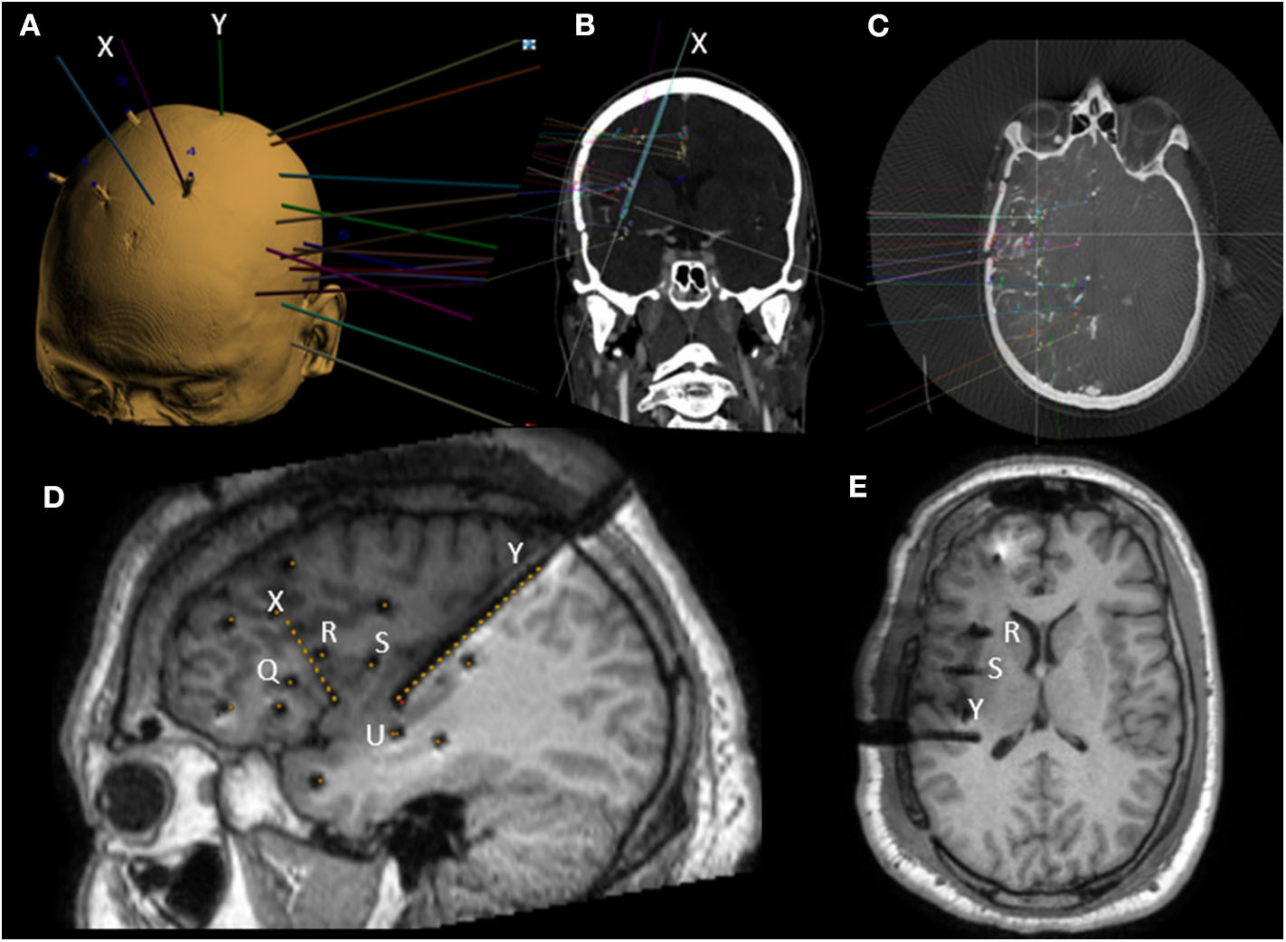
Example of preoperative SEEG planning on ROSA software and postoperative MRI with mixed orthogonal and oblique trajectories. (A) 3D view of a left SEEG planning along with bone fiducials markers. (B) In plane view along oblique anterior insular (X) trajectory. (C) Example of SEEG planning fused with 3D angiography. (D) Postoperative sagittal T1 MRI with dark shadows representing electrodes with yellow dots superimposed representing planned trajectories. Note the oblique trajectories (X and Y) and four orthogonal trajectories (Q, R, S, and U). (E) Postoperative axial T1 MRI with dark shadows representing electrodes. Note the end location of contacts in the upper insula of orthogonal electrodes (R and S).
